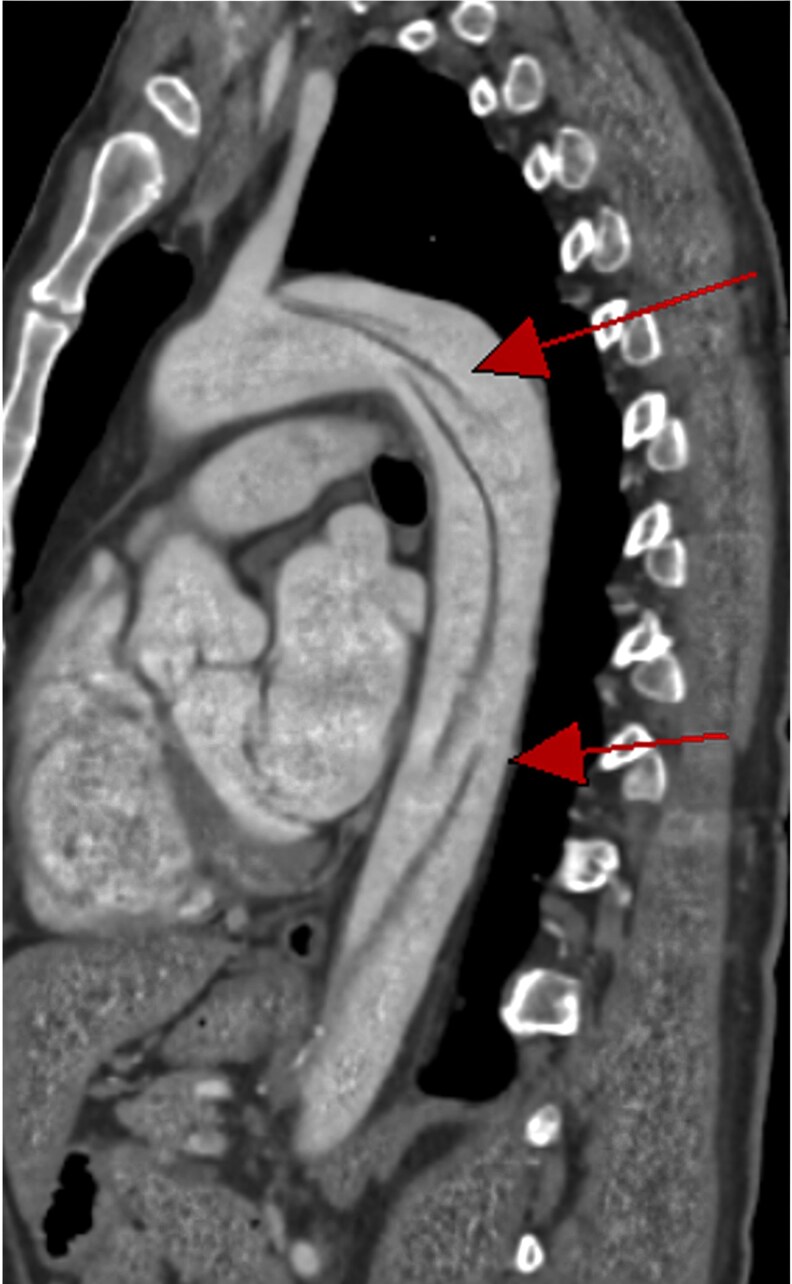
CTA of the thoracic aorta (sagittal section) showing a TBAD with two entry tears below the left subclavian artery (red arrows).
